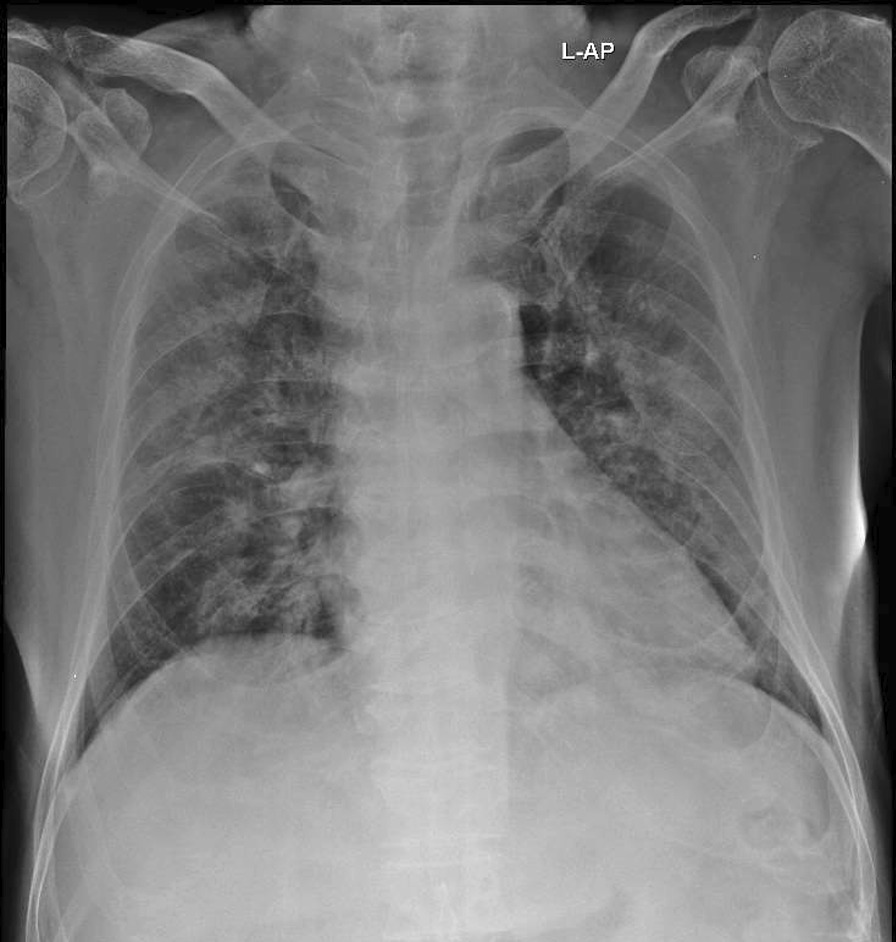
Chest X-ray showing bilateral pneumonia
